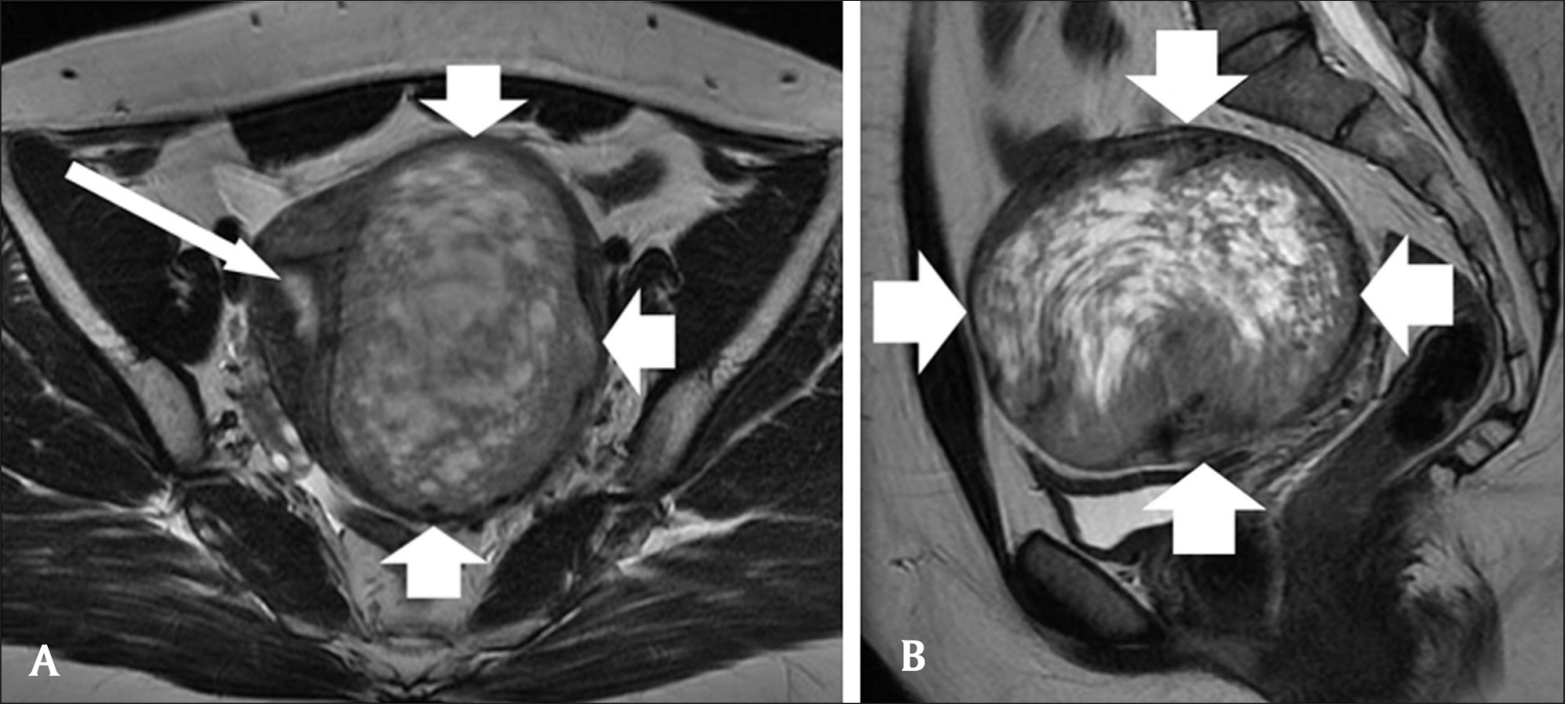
(A) Axial T2-weighted MR image: the hyperintense «snowstorm» ovoïd mass (thick arrows) and the uterine lumen (thin arrow). (B) Sagittal T2-weighted MR image: the tumor (arrows).
